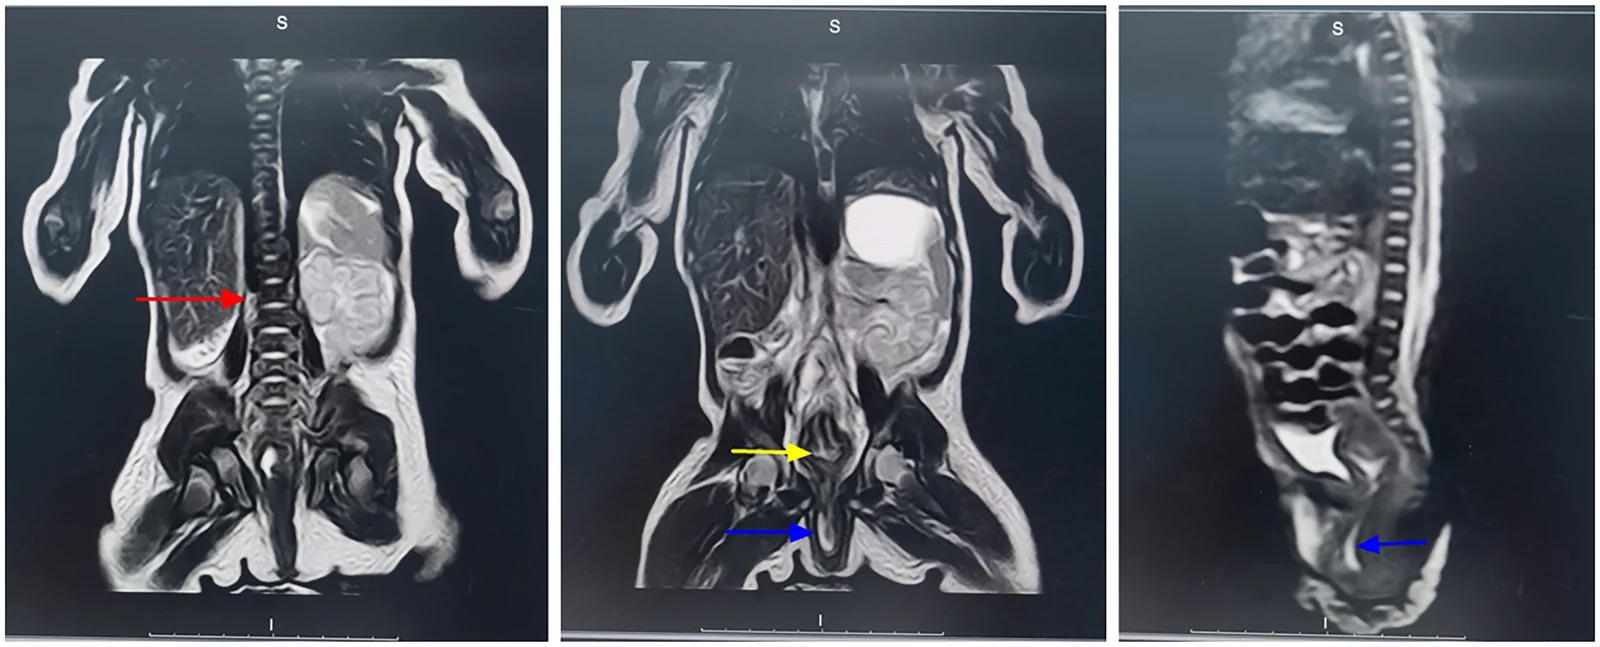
Magnetic resonance imaging (MRI) findings: right hypoplastic kidney (red arrow) (A); uterine malformation (bilateral uterine cavities and cervixes) (yellow arrow) (B); right vaginal fluid accumulation suggestive of vaginal septum oblique formation (blue arrow) (C).
